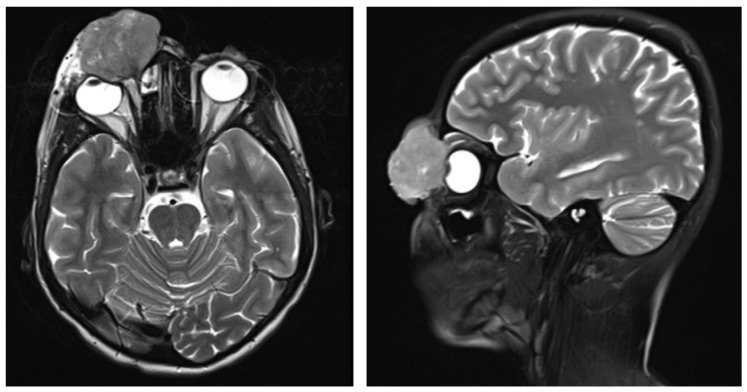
MRI of the orbits and brain (September 2024, 16 y.o.)—multinodular tumors, compressed eyeball and bones destruction.
